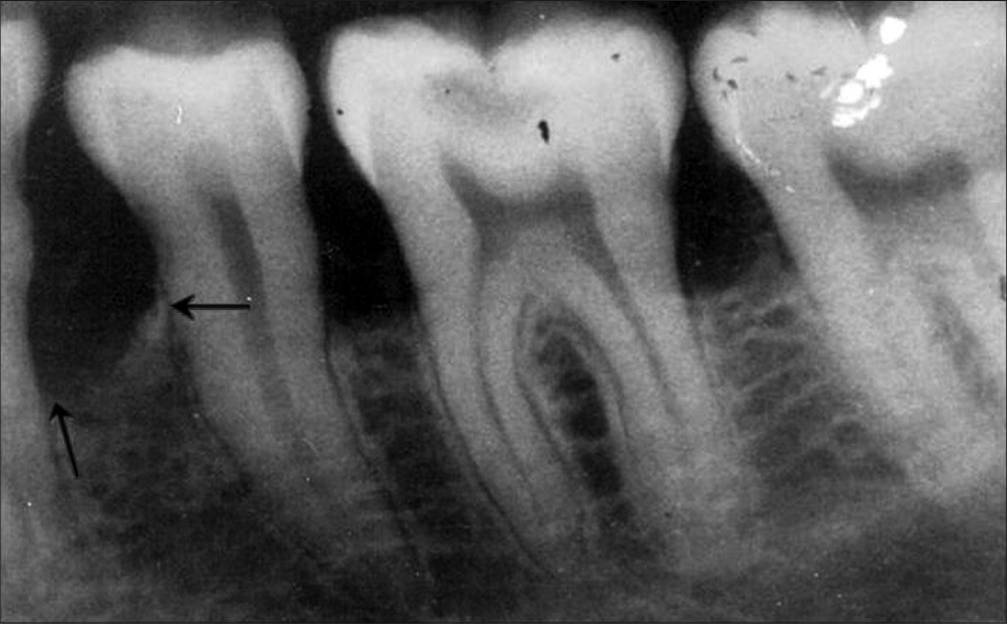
Pre-treatment periapical radiograph of mandibular left second premolar (tooth # 35) showing an inrabony defect on the mesial aspect
